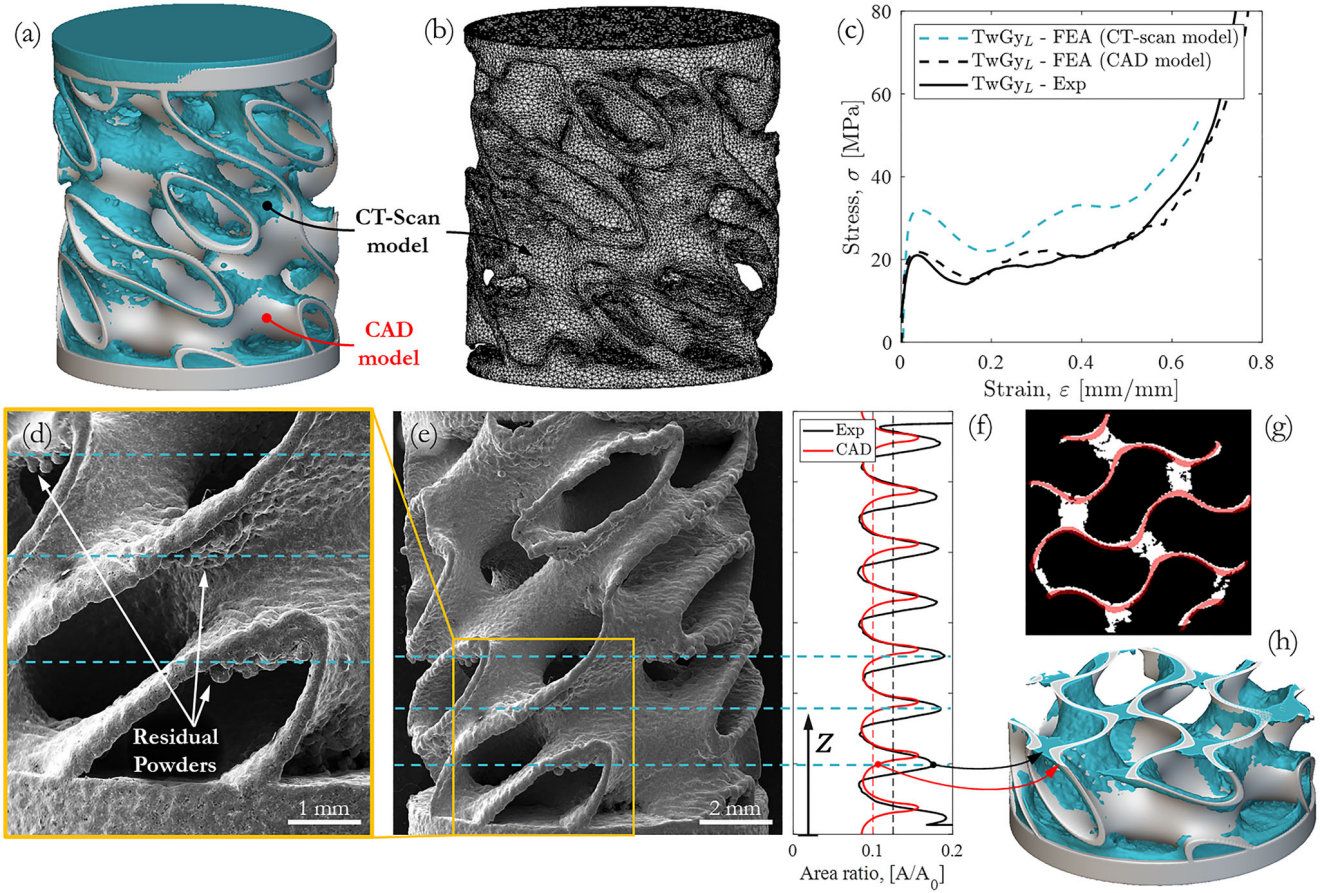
Effect of AM‐induced shape variations on the twisting gyroid structure. a) CT‐scan model superimposed on CAD geometry. b) Mesh generated from CT‐scan model. c) Experimental versus numerical compression response for the “TwGy_L_” (M=1) configuration. d,e) SEM images of gyroid wave surfaces. f) Area ratios along the build direction from CAD and CT‐scan models. g) Segmented layers of CAD and CT‐scan models. h) Comparison of CT and CAD geometry beneath a gyroid wave.
